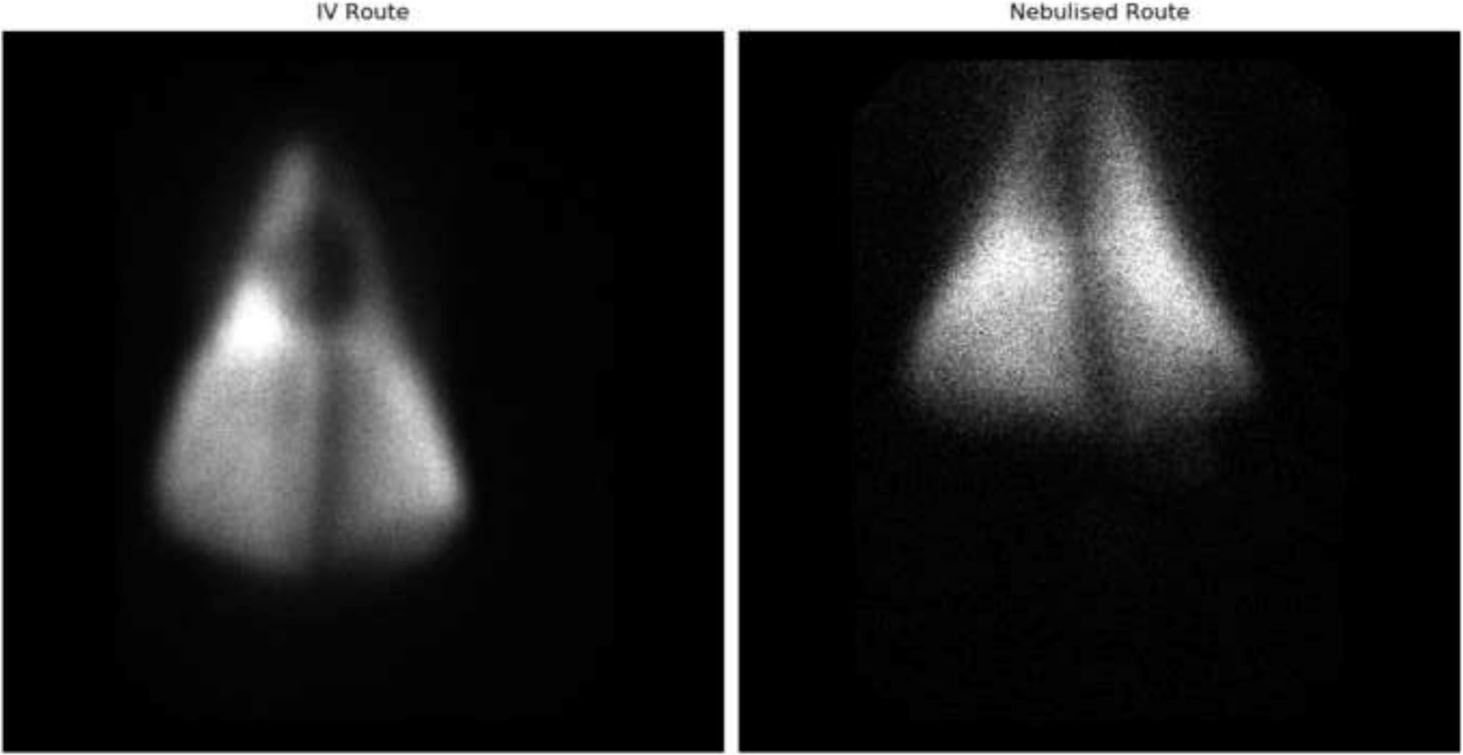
Comparative dorsal scintigraphy images of lung deposition of i.v and nebulised radiolabelled tobramycin
